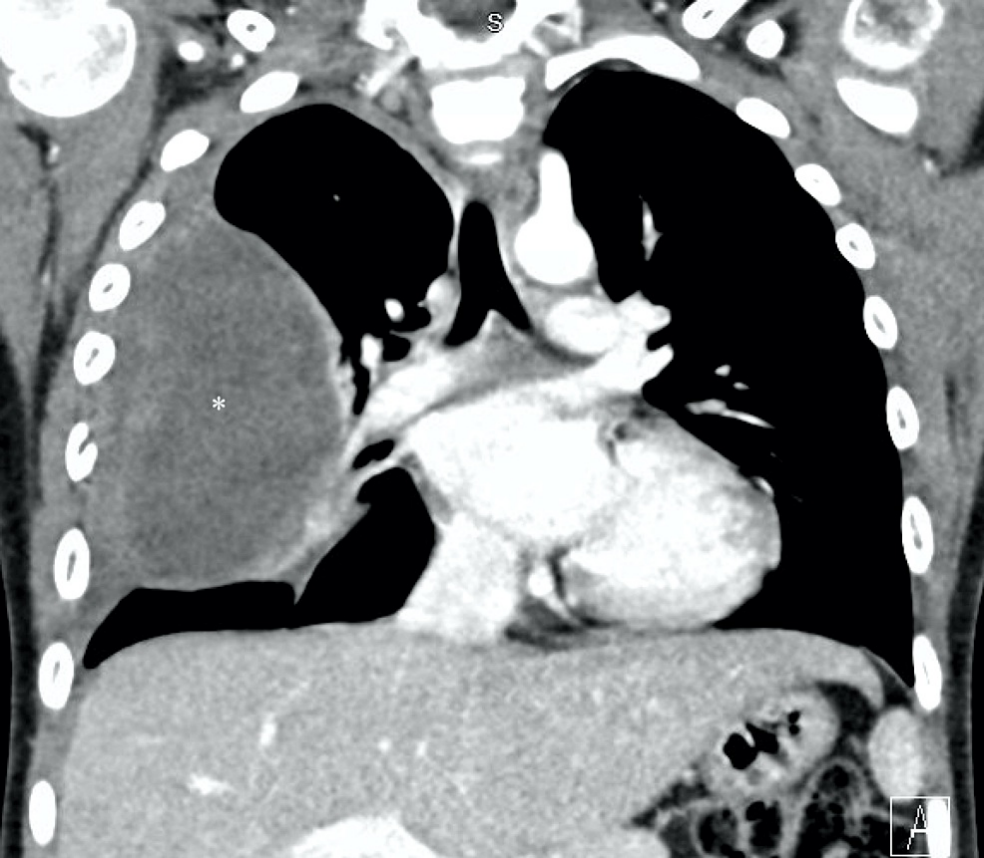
CT scan showing improved aeration of the right lung after six cycles of chemotherapy and shrinkage of the mass Mass now measuring 9.4x5.6x8.8 cm.
